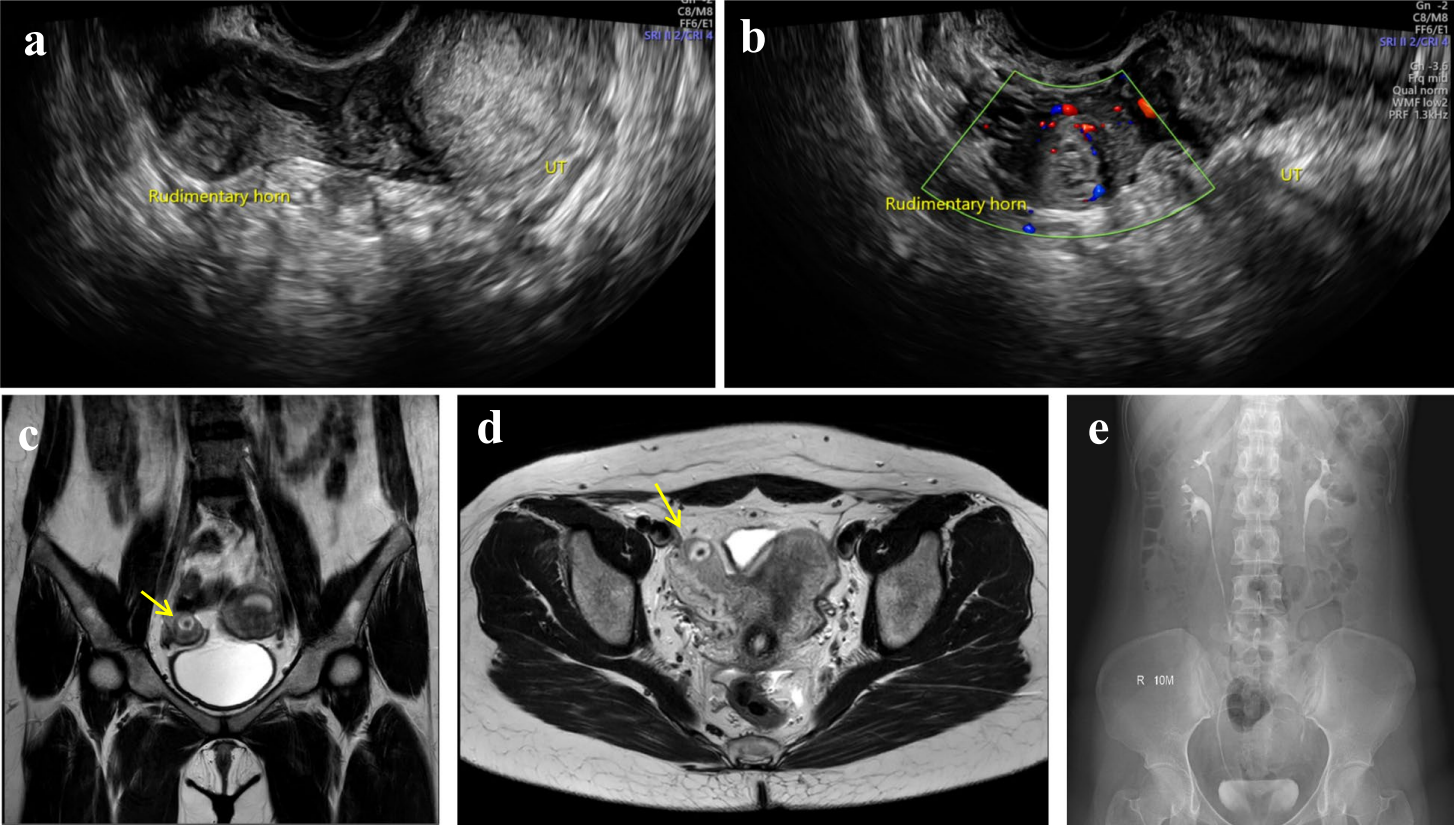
Transvaginal ultrasound (TVS) was examined, which diagnosed the left unicornuate uterus with the right rudimentary horn (a). Increased vascularity and double decidual sign suggesting right rudimentary horn pregnancy (b). T2-weighted Magnetic resonance imaging (MRI) of the pelvis showed about 8 mm-sized relatively thick-walled cystic lesions at the cornus of the right rudimentary horn or right salpinx proximal portion, suggesting RHP (c and d). Intravenous pyelogram revealed right duplex kidney (e)
